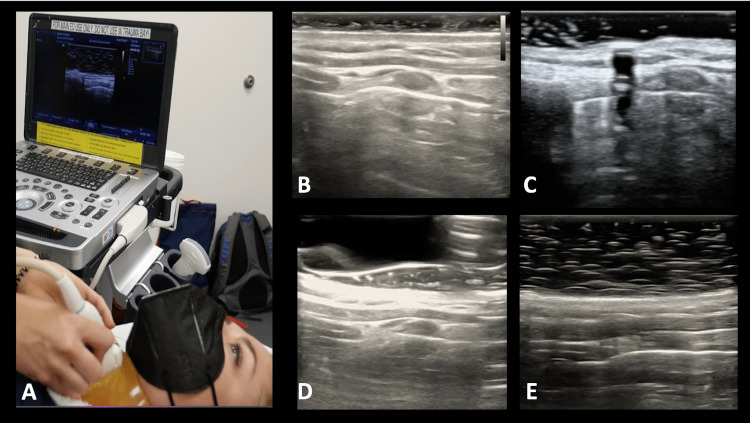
Representative images of (A) examination set-up with gel model placement over the anterior neck, (B) ultrasound of normal thin anterior neck model, (C) ultrasound of neck model with subcutaneous air, (D) ultrasound of model with hematoma or fluid collection over the anterior neck, (E) ultrasound of thick, obese neck model
